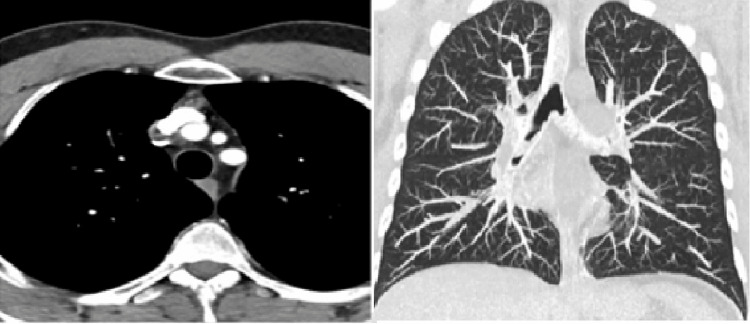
CT-chest with contrast shows normal lung volume and thymus gland.
